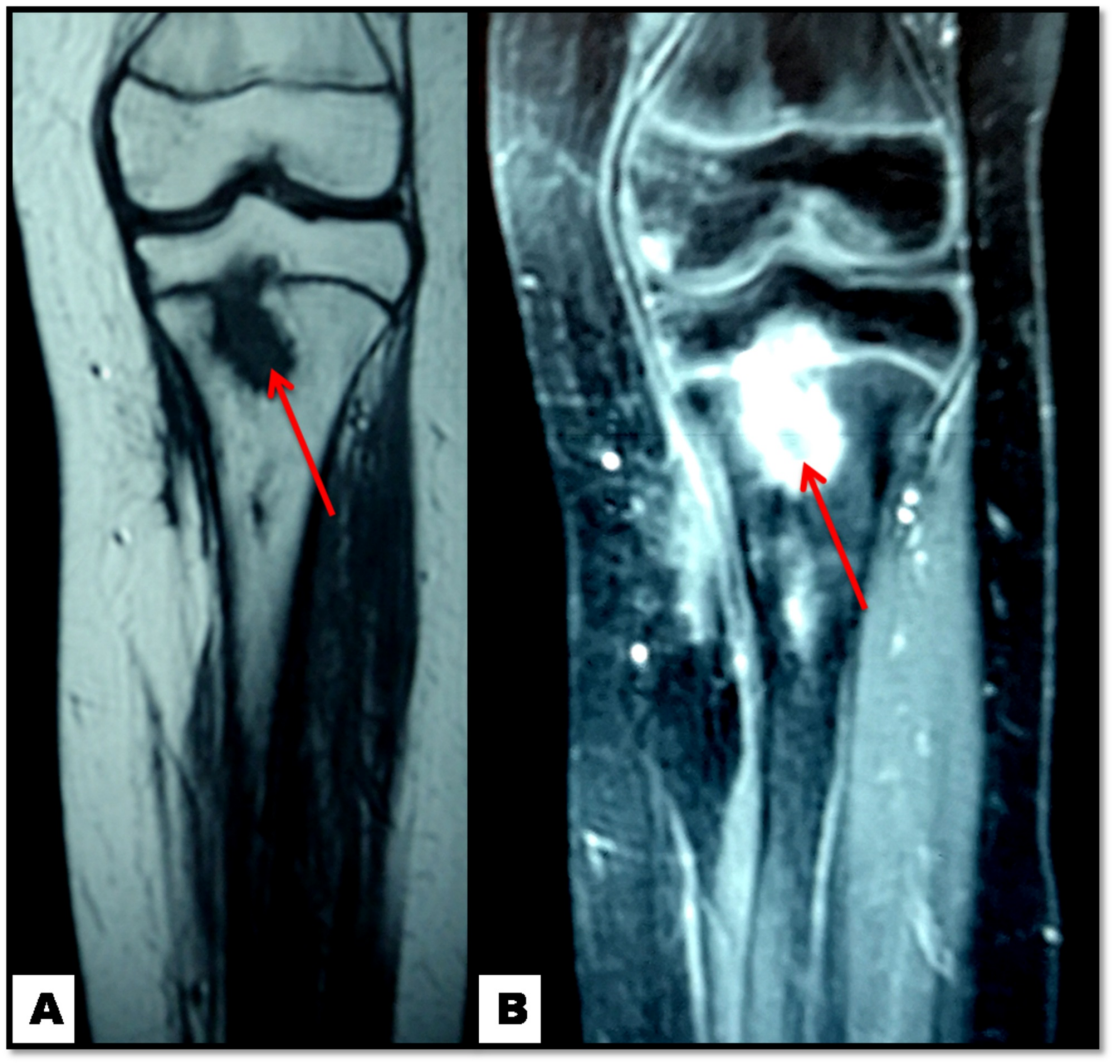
Knee MRI: osteomyelitis of the proximal tibia (red arrows) at six months follow up A: coronal T1-weighted sequence; B: coronal T2-weighted sequence
